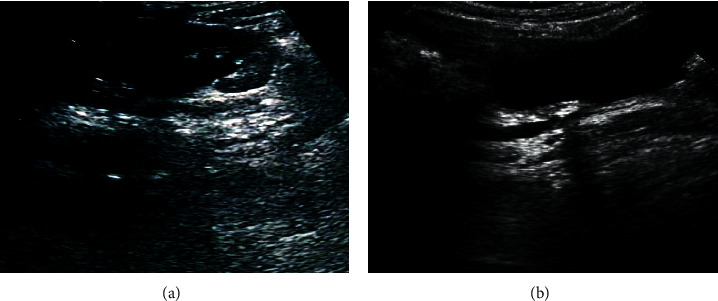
Ultrasound images of the patients: (a) an ultrasound of a child with melamine urinary calculi; (b) an ultrasound of a child with melamine urinary calculi and renal failure.
